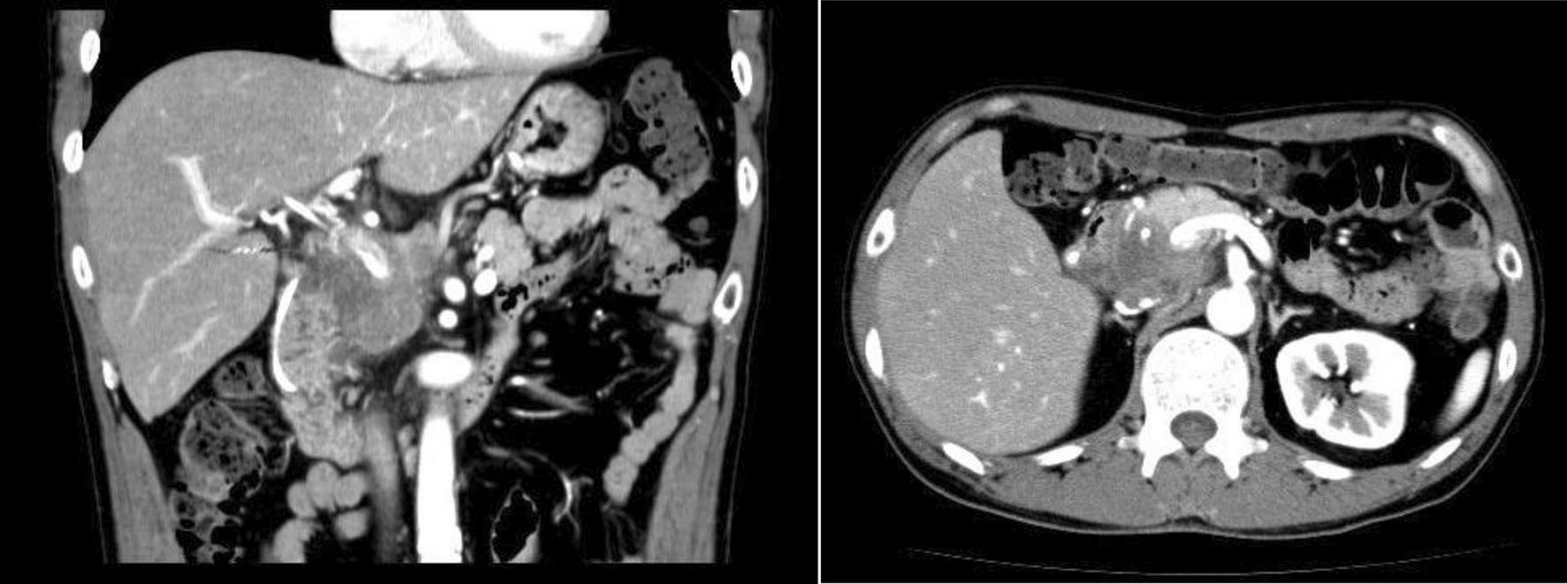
Contrast-enhanced abdominal CT shows tumor involvement in the portal vein and inferior vena cava and soft irregular tissues surrounding the celiac artery
